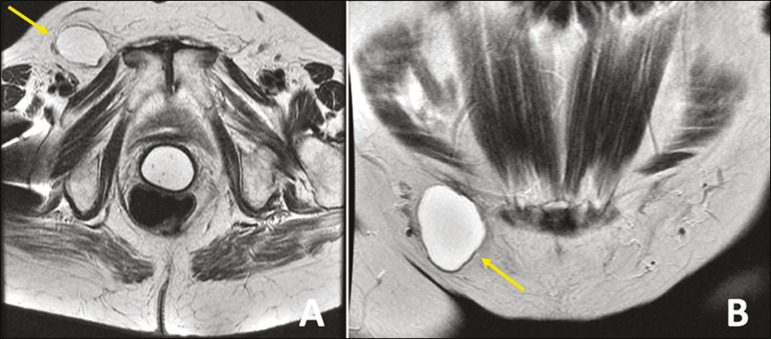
Axial and coronal T2-weighted MRI sequences (A and B, respectively) showing a lesion with a cystic aspect in the right inguinal canal, with thin walls, no septa, and no solid components, consistent with a cyst of the canal of Nuck. Image courtesy of Dr. Fernando Mansano.
